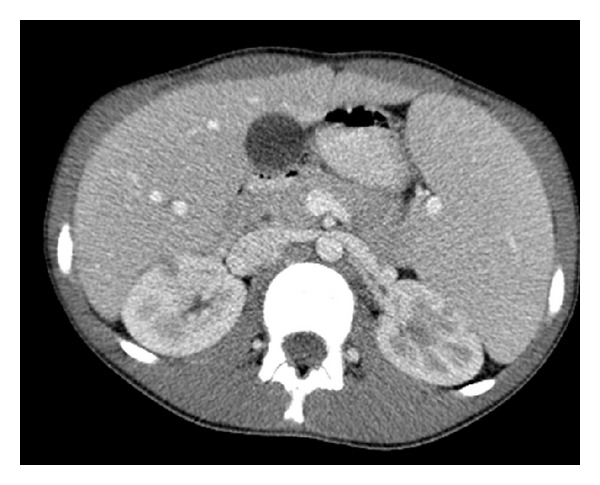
Axial contrast enhanced CT shows multiple, small, bilateral hypodense kidney lesions in keeping with lymphomatous involvement.
